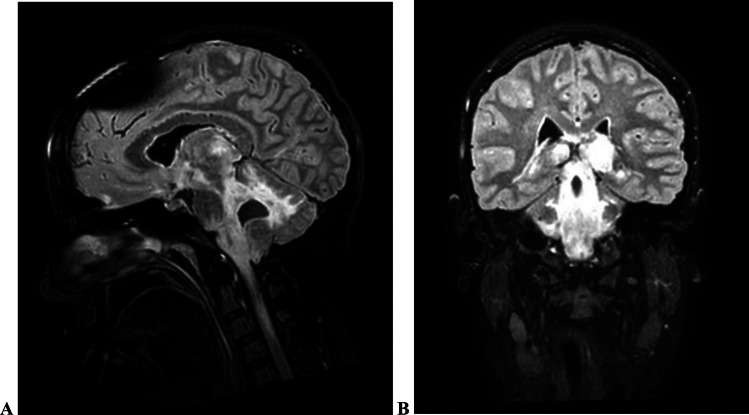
Image A is the sagittal T2-weighted brain MRI with FLAIR-sequence showing the extensive changes from the brainstem all the way to C2. Image B is the coronal diffusion weighted image showing the extent of the changes
